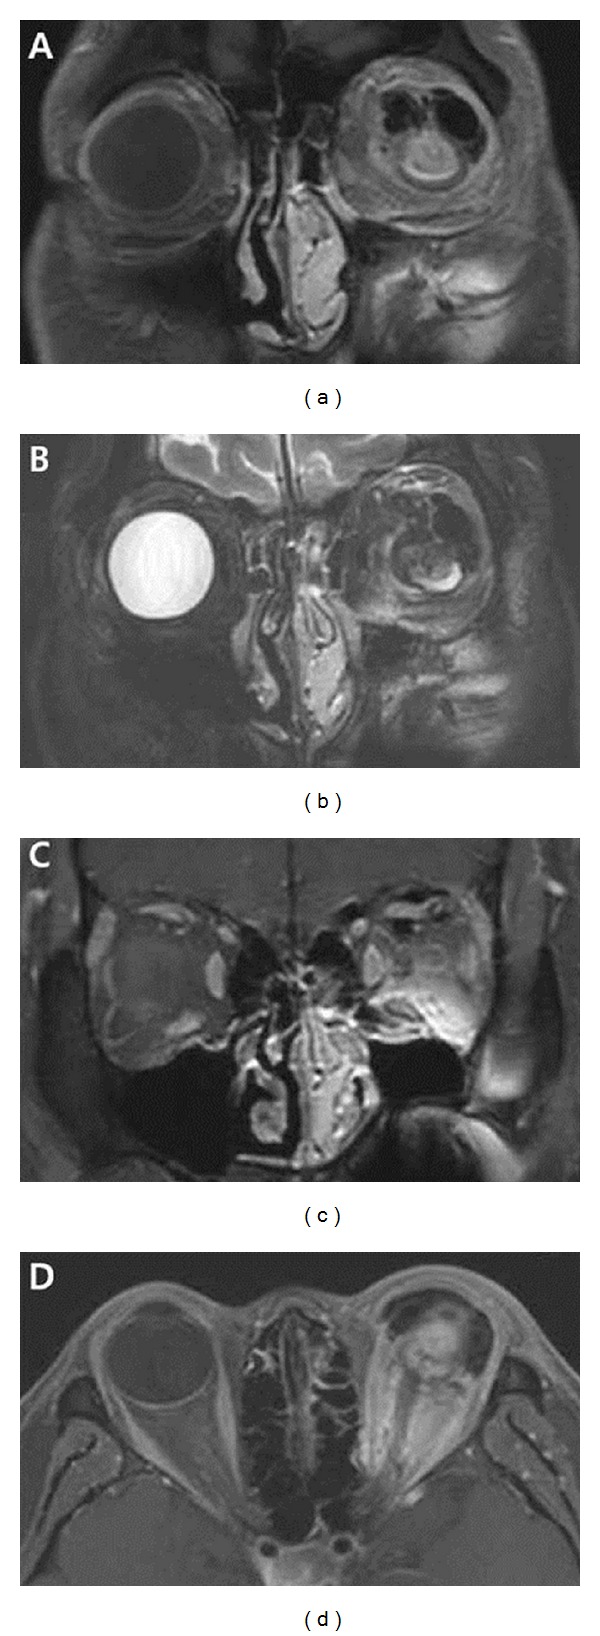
Magnetic resonance image (MRI). (a), (b) T1- and T2-weighted images reveal a large septate cystic mass in subconjunctival and orbital space with low signal intensity. (c), (d) The mass is located in the superior orbit accompanied by inflammatory reaction showing heterogenous enhancement in Gd-enhanced T1-weighted images.
